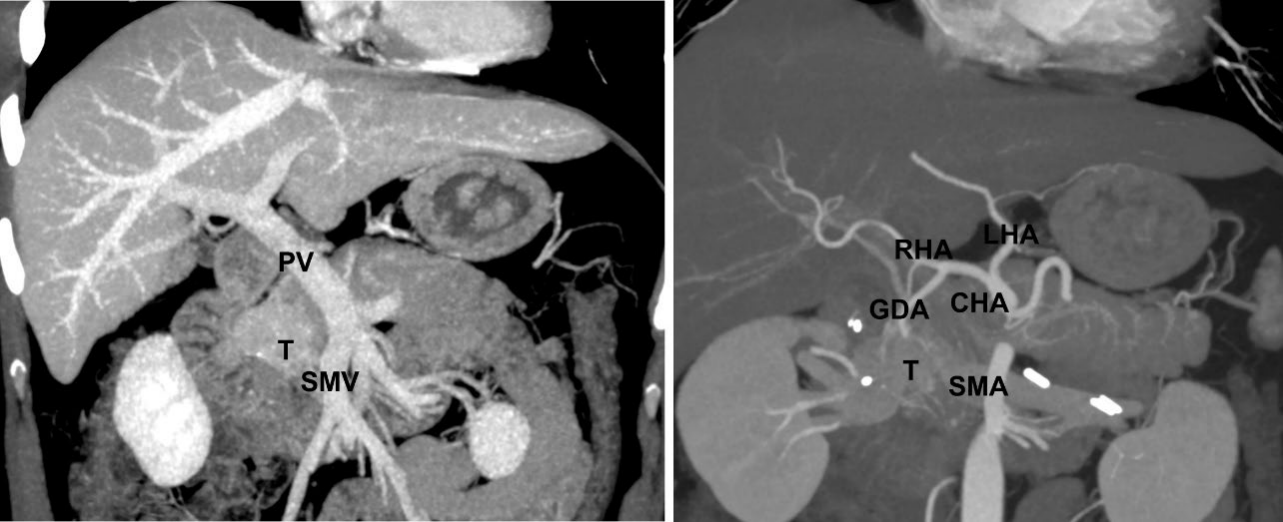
Angiotomography computed tomography scan. Venous and arterial reconstructions. The patient has an uncommon replaced gastroduodenal artery arising from the right hepatic artery, which not interfere with surgical planning. (T) tumor; (PV) portal vein; (SMV) superior mesenteric vein; (CHA) common hepatic artery; (SMA) superior mesenteric artery; (RHA) right hepatic artery; (LHA) left hepatic artery; (GDA) gastroduodenal artery.
